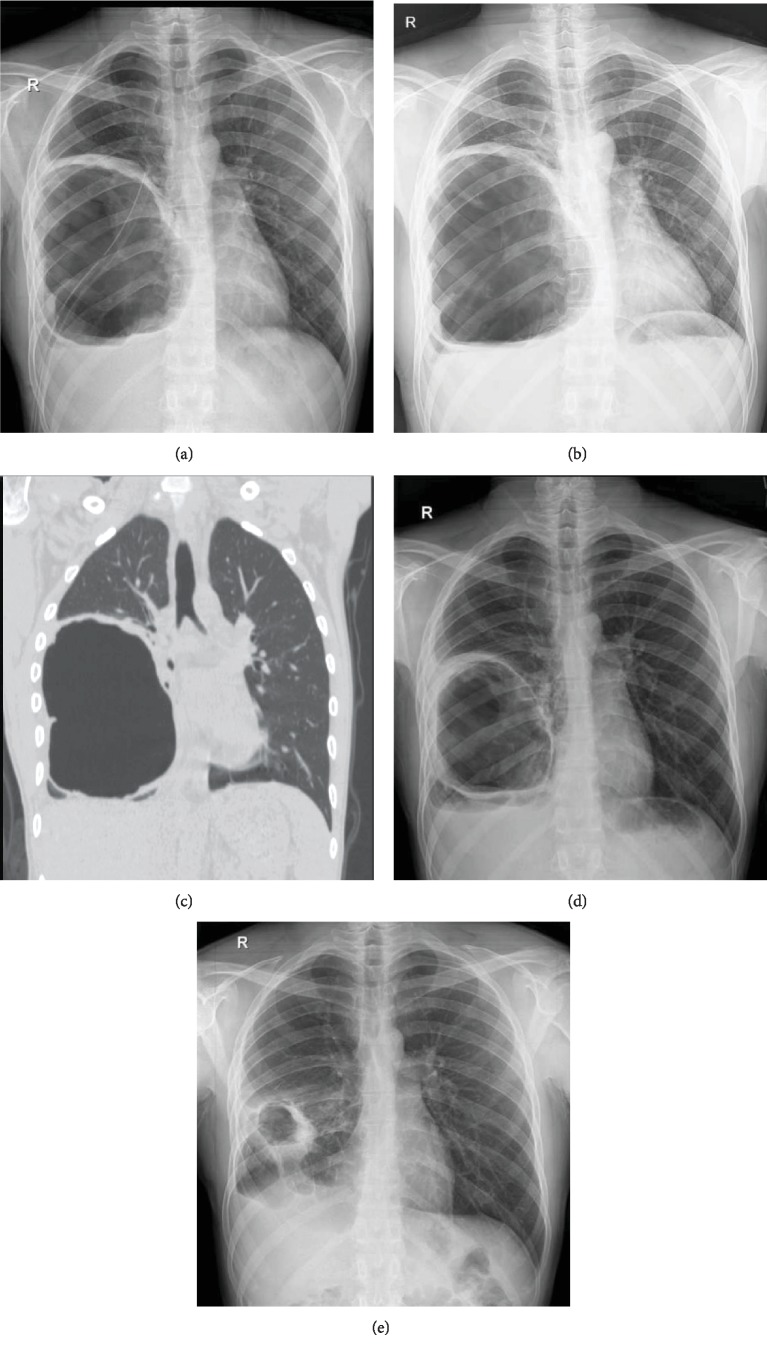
Postoperation radiography of a pulmonary hydatid cyst with lung-preserving surgery: (a) postop; (b) on discharge; (c) chest CT 2 months after surgery; (d) after a 6-month follow up; (e) after a 12-month follow up.
